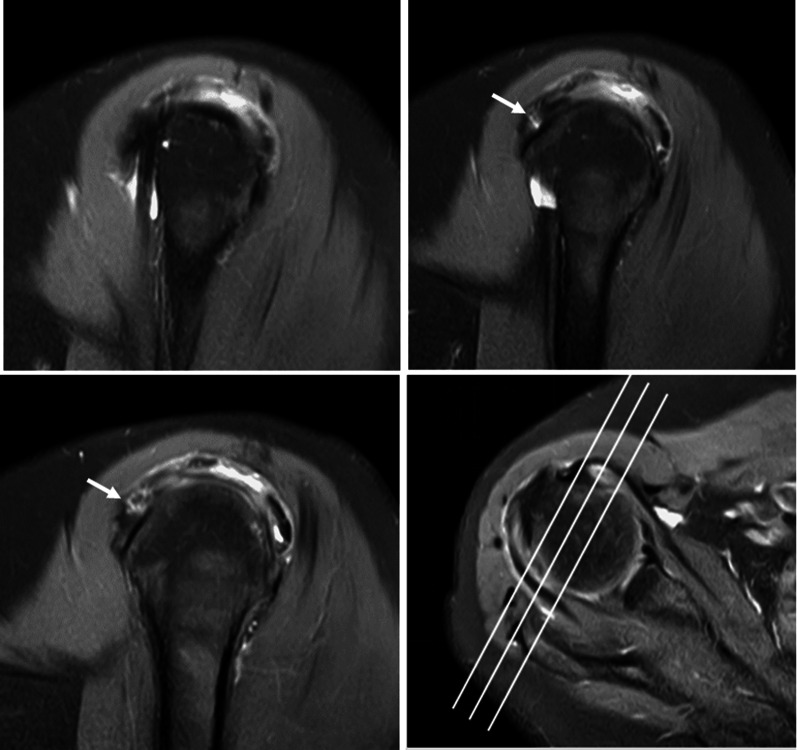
Type-B of fissure sign. There is a vertical fluid-filled gap into the tendon involving both sides surface of SSC at the continuous two slices on oblique sagittal IM-WI/fs MRI counted from the most lateral cortex of lesser tuberosity to the medial SSC footprint area referring to the axial MR image (white arrow)
